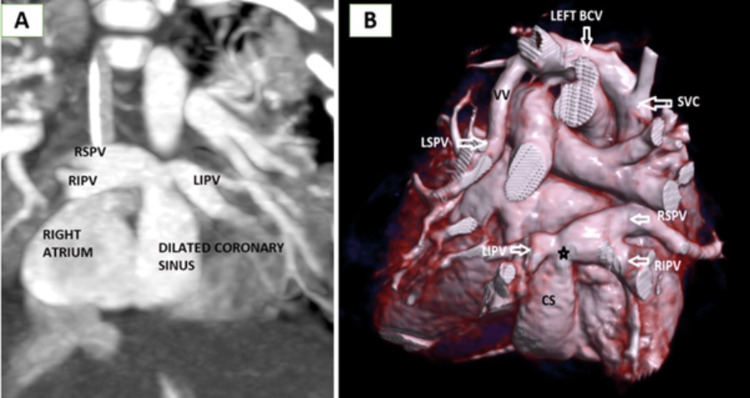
Mixed TAPVC (A and B) cardiac CT coronal MIP (A) and 3D VR (B) image showing RSPV and RIPV forming confluence with LIPV and joining the dilated CS and (B) coronal 3D reconstruction showing intracardiac drainage of the RSPV and bilateral inferior pulmonary veins draining into the dilated CS and LSPV coursing cranially to drain into the LEFT BCV via a VV, resulting in both supracardiac and intracardiac anomalous pulmonary venous connections TAPVC: total anomalous pulmonary venous connection, MIP: maximum intensity projection, CT: computed tomography, VR: volume rendered, RSPV: right superior pulmonary vein, RIPV: right inferior pulmonary vein, LIPV: left inferior pulmonary vein, LSPV: left superior pulmonary vein, CS: coronary sinus, LEFT BCV: left brachiocephalic vein, VV: vertical vein
